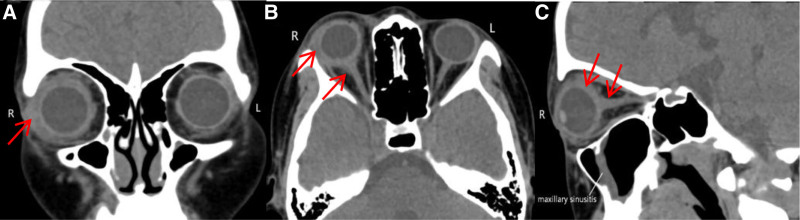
Computed tomography scans (coronal, A; axial, B; and sagittal views, C) of the same patient demonstrating evidence of the right maxillary sinusitis, marked thickening of the right eye and as optic nerve along its orbital track.
